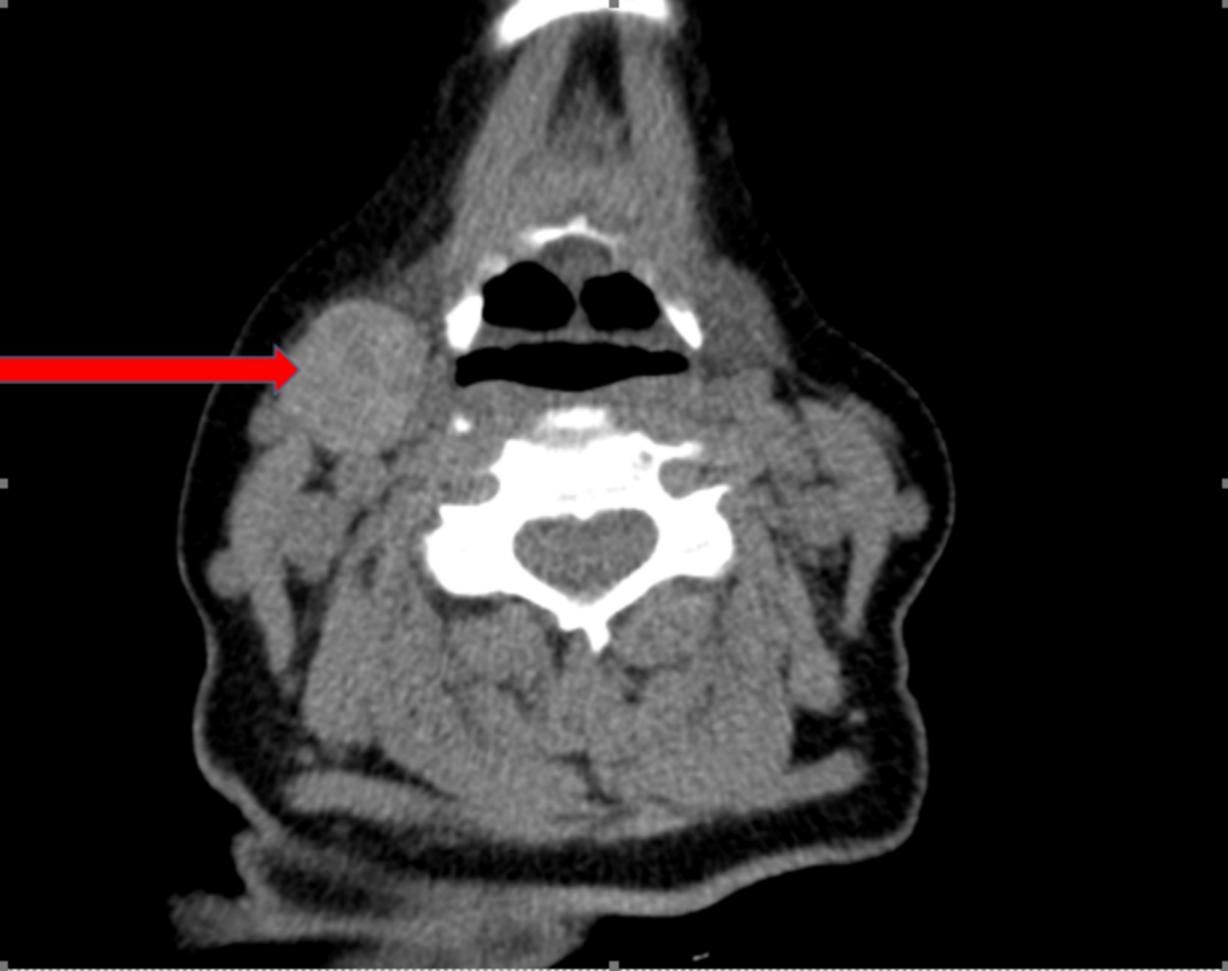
Computed tomography of the neck Computed tomography of the neck demonstrating the location of the ectopic thyroid tissue (Red arrow).
